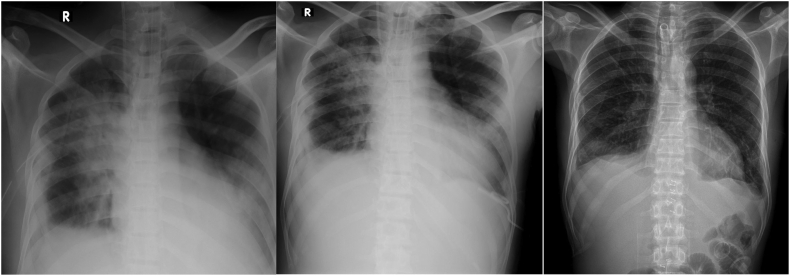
Series of chest radiograph showing improvement following thoracoscopic drainage (VATS) and decortication of the lungs showing.
